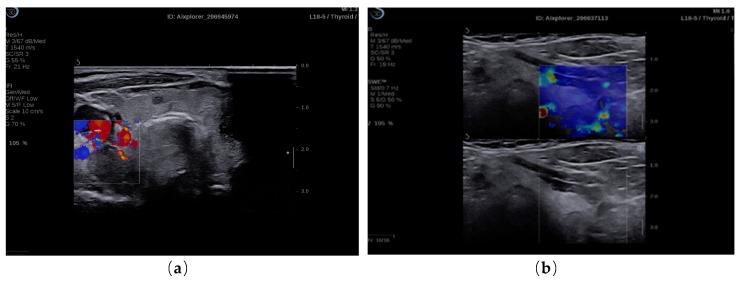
(a) 2 B-mode ultrasound evaluation of parathyroid hyperplasia, visualizing parathyroid hyperplasia in color dopple-r mode; (b) elastogram overlapping B-mode image of parathyroid hyperplasia, color map of tissue elasticity.
